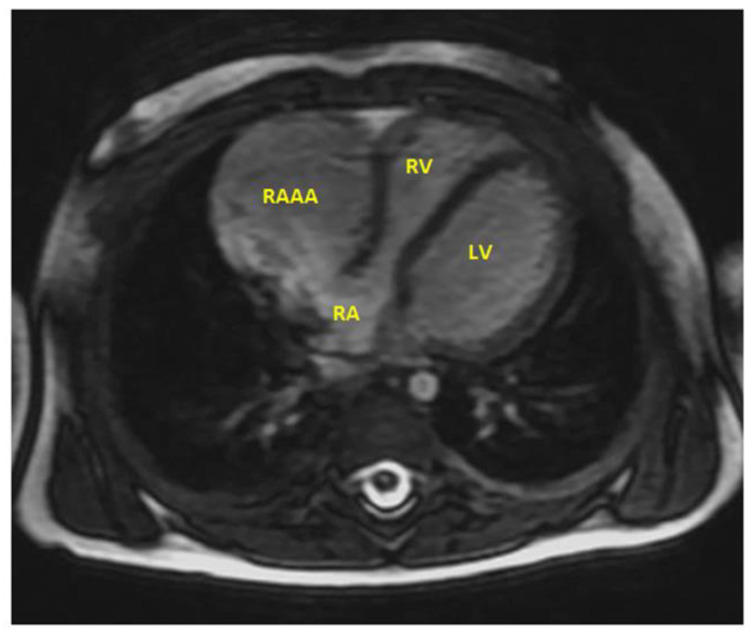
MRI in a ten-month-old infant. A gigantic aneurismal dilatation of the RAAA was detected.
